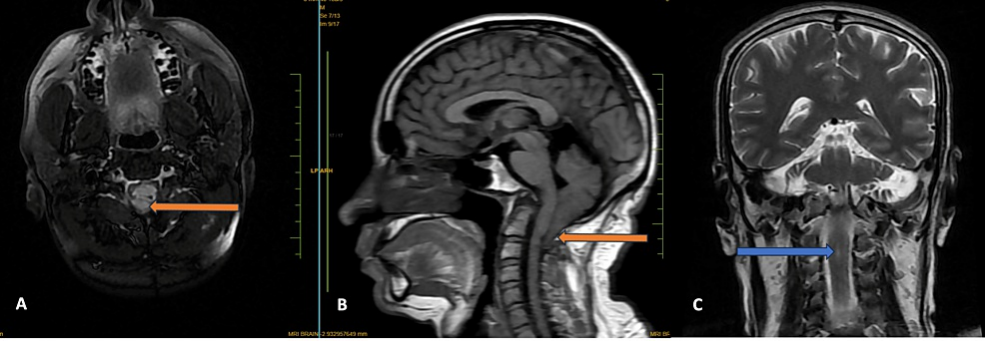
MRI of the brain Magnetic resonance imaging (MRI) of the brain, axial T2 fluid-attenuated inversion recovery (FLAIR), and mid-sagittal T1-weighted images show tonsillar herniation (orange arrow) (A and B). The mid-sagittal T1-weighted image also shows platybasia with an abnormal skull base angle of 147 ° degrees (B). The coronal T2-weighted image shows a small syrinx at the C2-C3 intervertebral disc level (blue arrow) (C).
